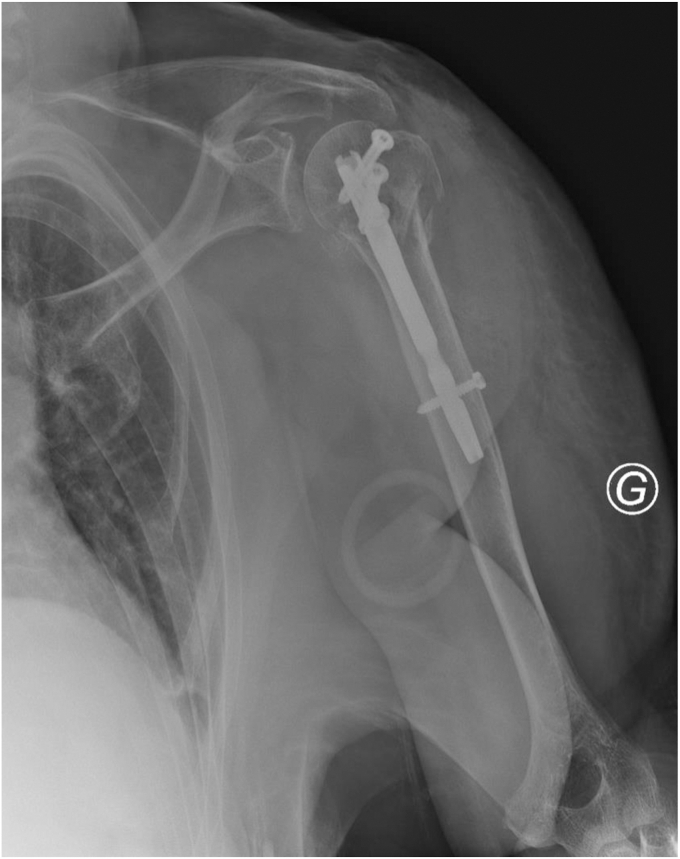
Radiographic assessment after closure.
